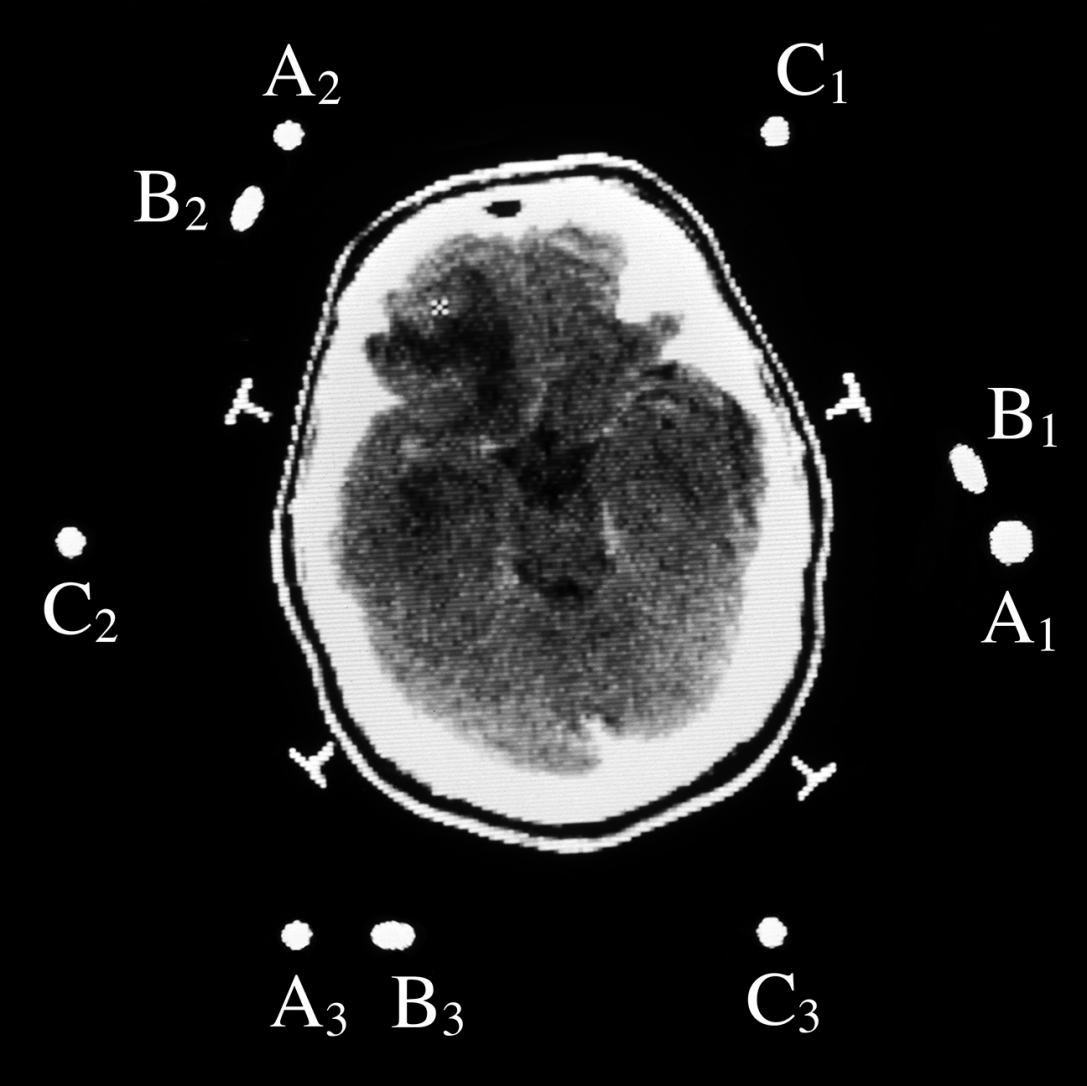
CT Image with Three Sets of Fiducials CT image of a patient to whom a BRW CT localizer frame (Integra Radionics Inc., Burlington, MA), which comprises three N-localizers, is attached. The cross sections of the three N-localizers create three sets of fiducials \begin{document}\left\{ \mathrm{A_1,B_1,C_1} \right\}\end{document}, \begin{document}\left\{ \mathrm{A_2,B_2,C_2} \right\}\end{document}, and \begin{document}\left\{ \mathrm{A_3,B_3,C_3} \right\}\end{document} in the CT image. The cursor indicates the target point \begin{document}P_T\end{document}. The large vertical rod \begin{document} \mathrm{A_1} \end{document} allows it to be unambiguously distinguished from the other vertical rods and provides a visual cue that this figure is rotated approximately 90 degrees clockwise relative to Figure 6 [6].
